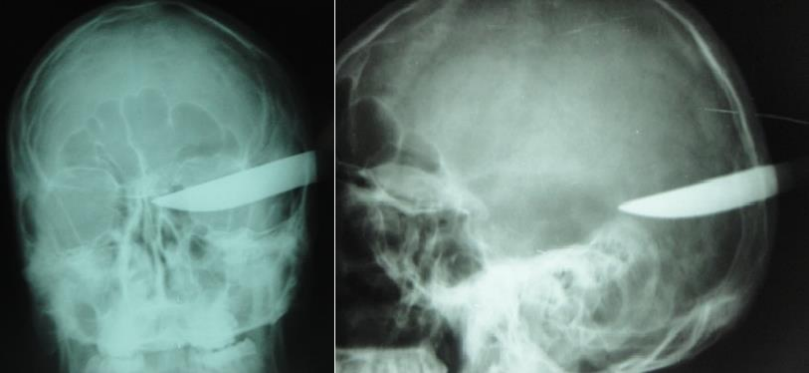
Skull X-ray shows the knife penetrating the skull
